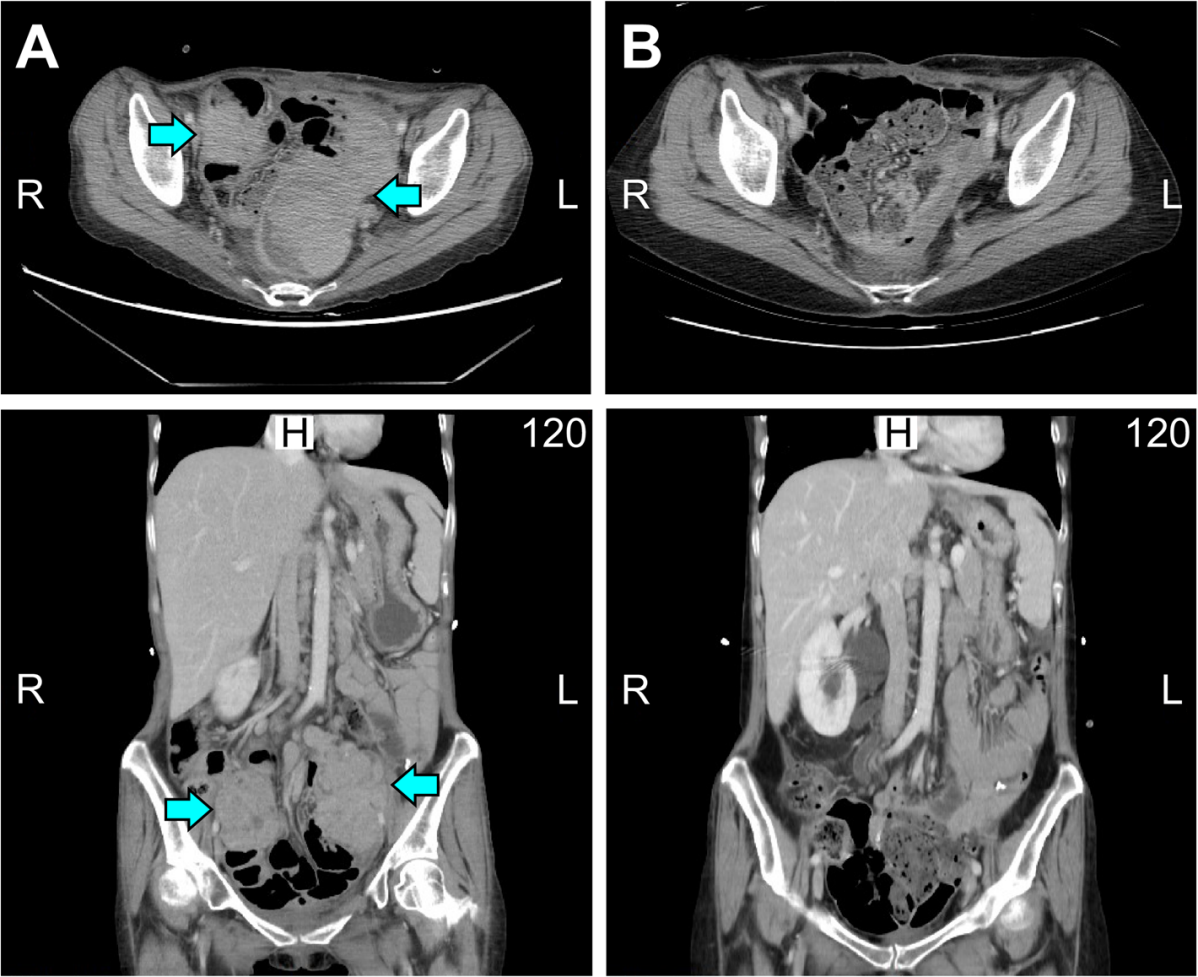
CT scan before and after the dual combination therapy. a Recurrent pelvic tumors (yellow arrow) were noticed in May 2019 (upper panel: axial view; lower panel: sagittal view). b Significant regression of recurrent pelvic tumors was found in November 2019 (upper panel: axial view; lower panel: sagittal view)
